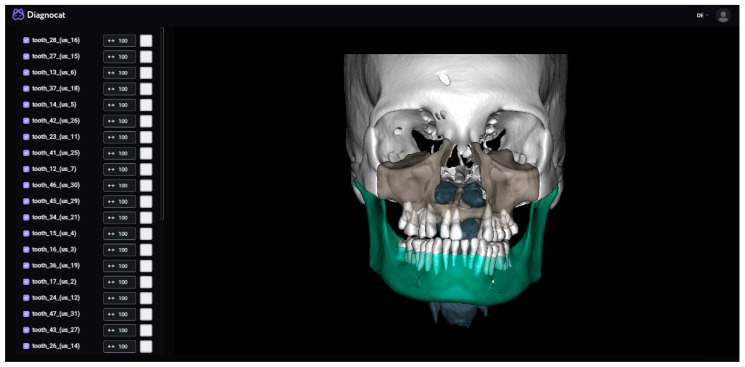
Diagnocat’s user interface (CT w/A 1 displayed).
